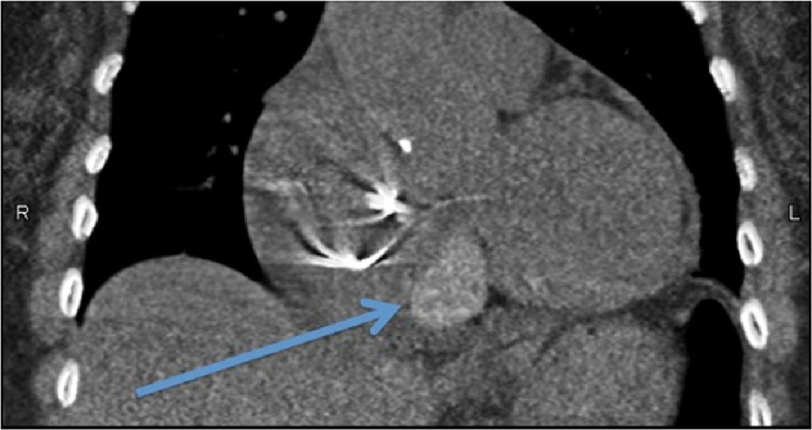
The mass was located at the anteroinferior aspect of the interatrial groove and to the right of the coronary sinus, as indicated by the arrow on this coronal cut of the patient’s cardiac CT for calcium scoring. Also visualized are her pacemaker leads.
